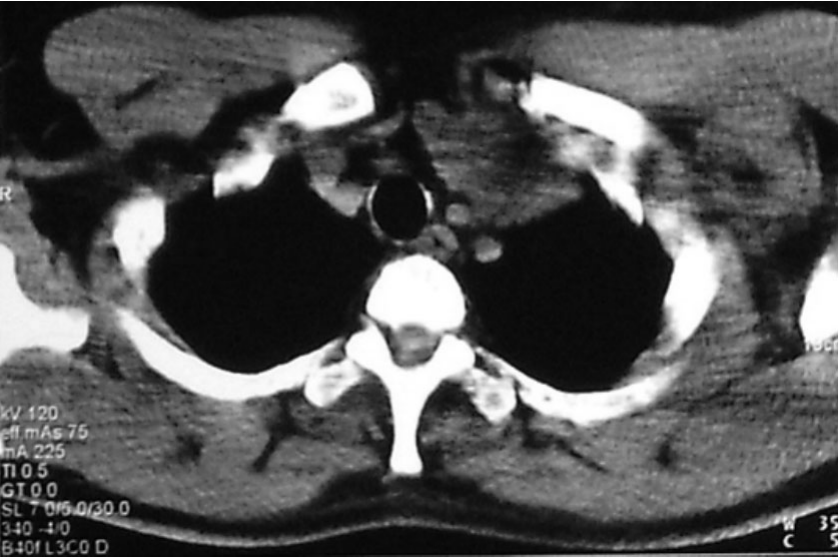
Computed tomography scan showing cervical mass with extension to anterior mediastinum in 2005.
